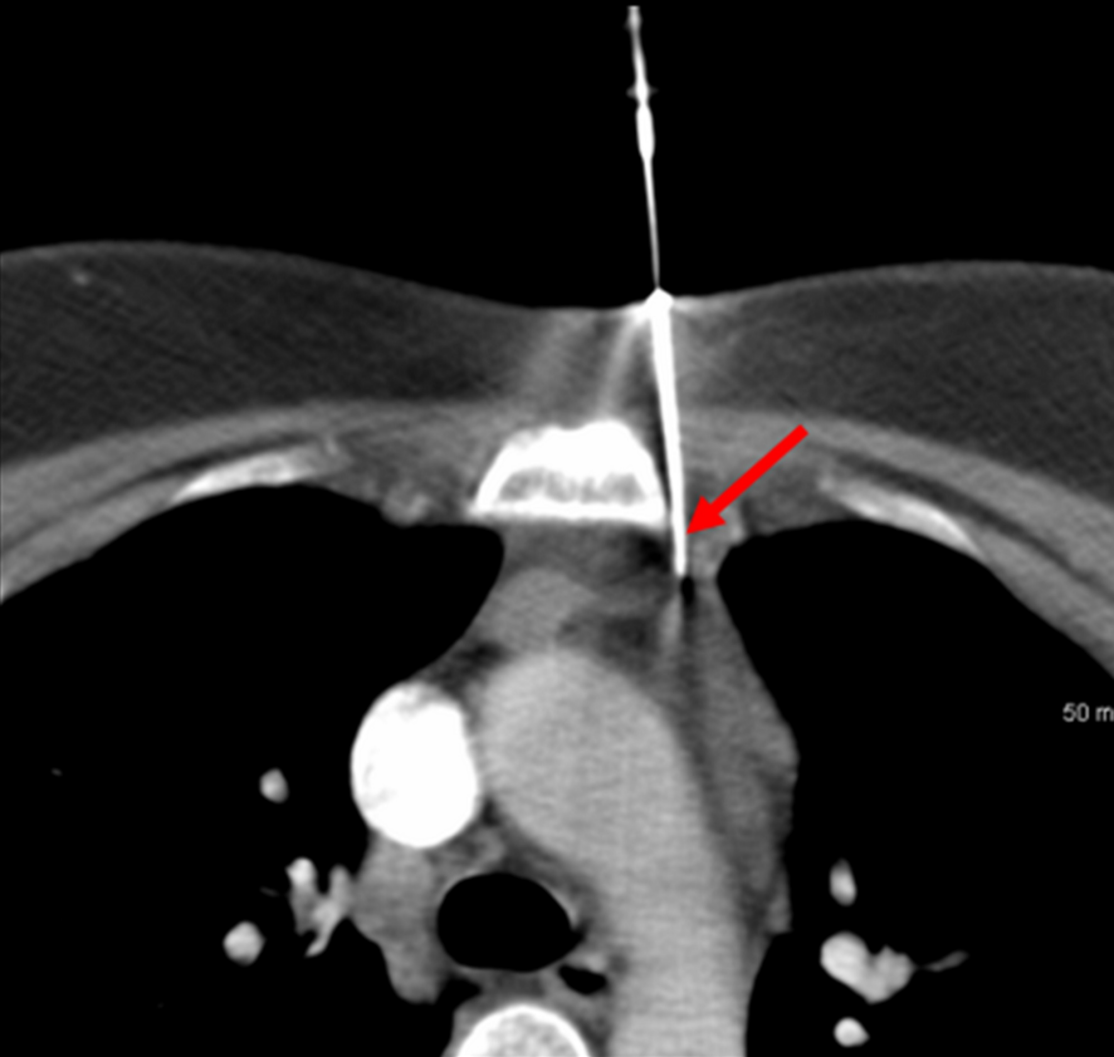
Biopsy of the Anterior Mediastinal Mass Using a Parasternal Approach The biopsy needle device was inserted just lateral of the left sternum using a parasternal approach (red arrow). This was determined to be the safest and most effect means for biopsying the anterior mediastinal mass.
